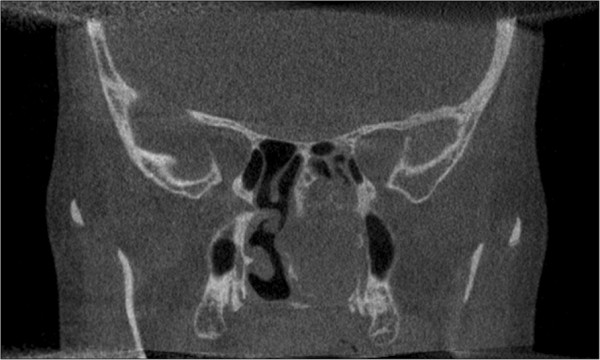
Coronal non-contrasted computed tomography scan of the skull, showing a tumorous lesion in the left nasal cavity extending 3.7 × 3.2 × 2.6 cm in diameter with partial infiltration of the surrounding structures.
